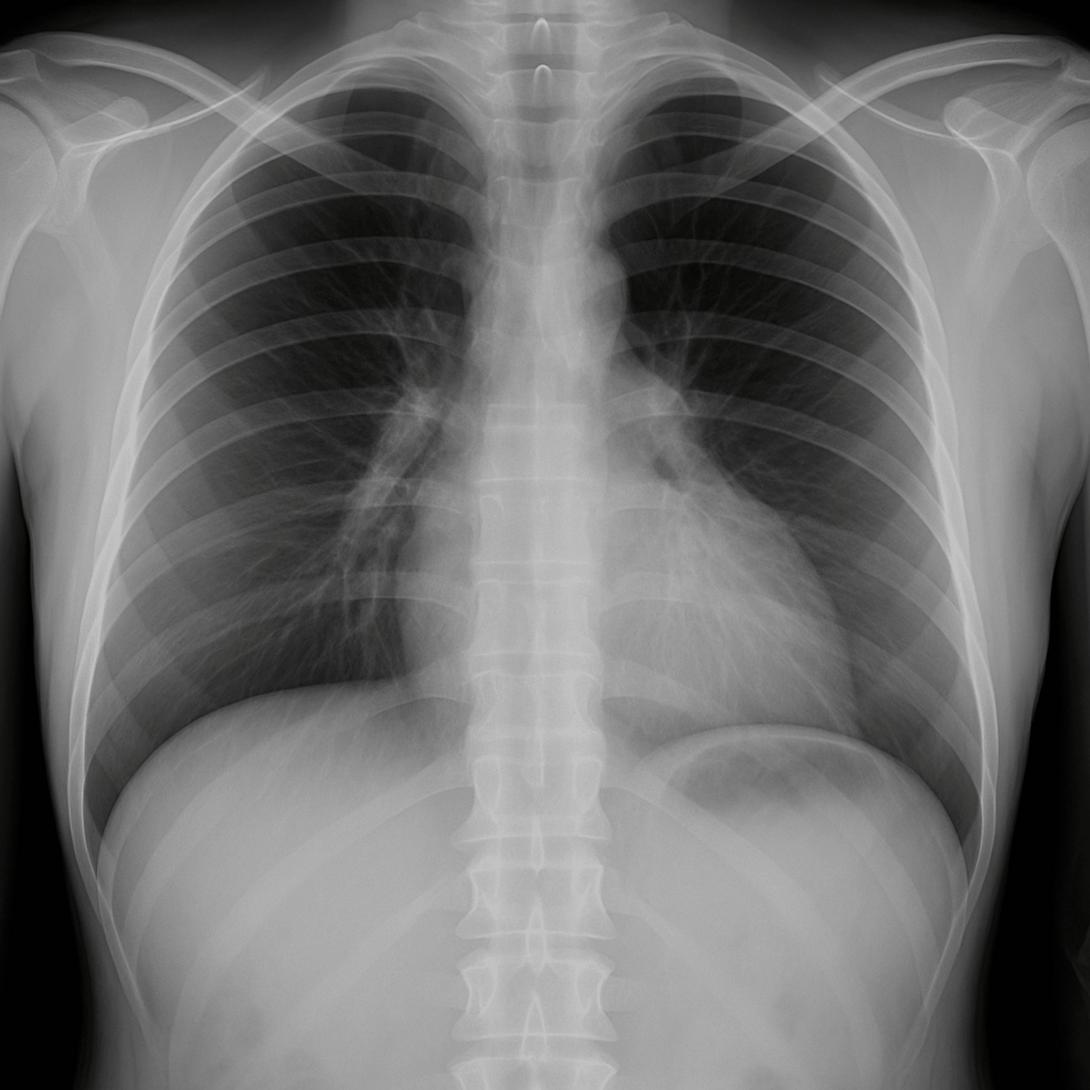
Chest X-ray showing hyperinflated lungs with mild hilar congestion
